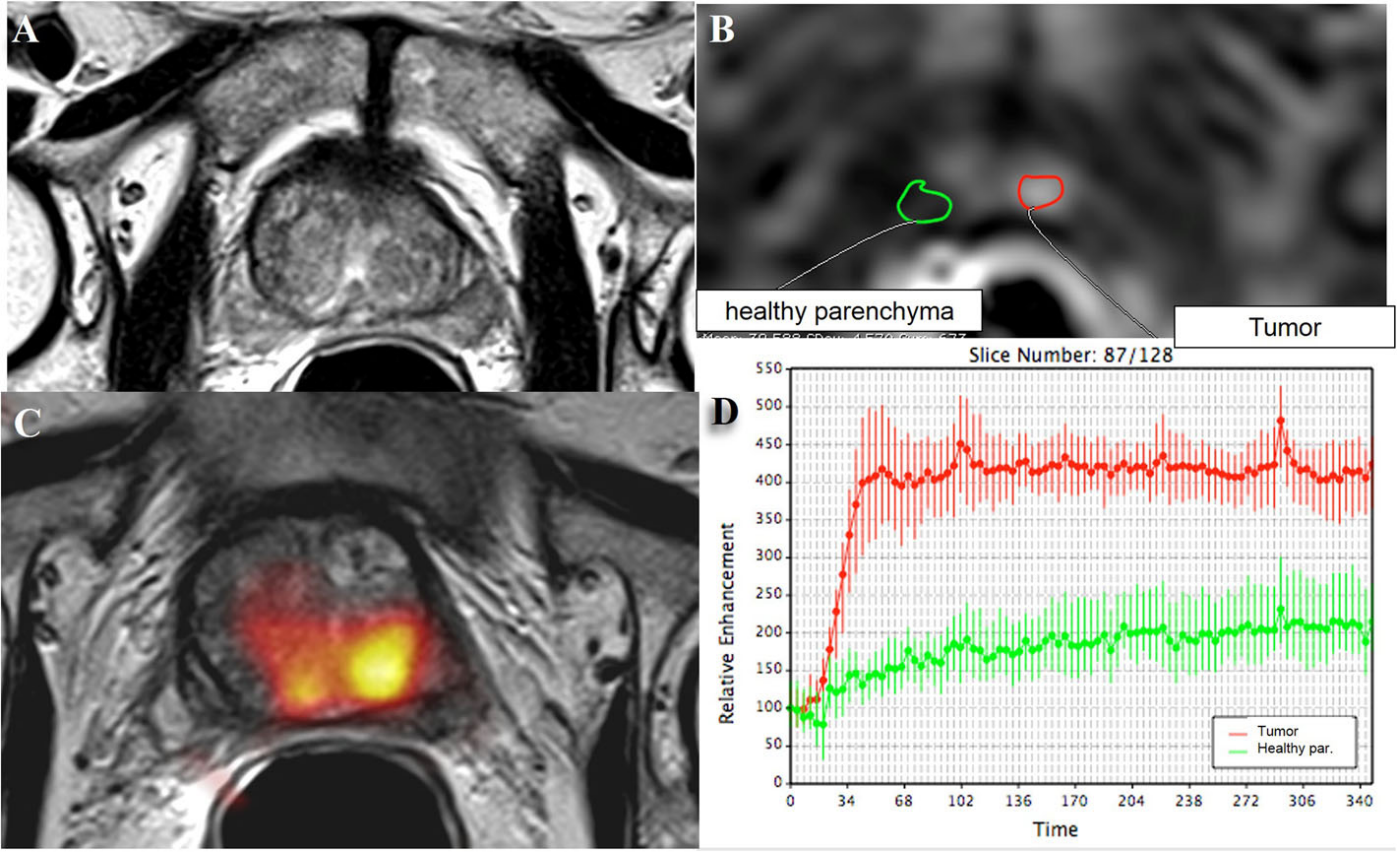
DCE-MRI (a, b, c) with time intensity curve (d) for tumor area and healthy parenchyma area
